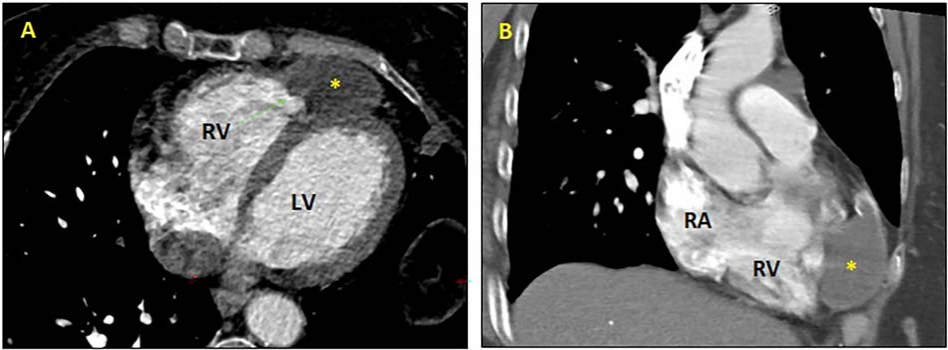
CT scan: axial (A) and sagittal (B) views showing hydatic intrapericardial cyst (yellow star) on the right ventricle’s apex. RA: right atrium; RV: right ventricle; LV: left ventricle.
